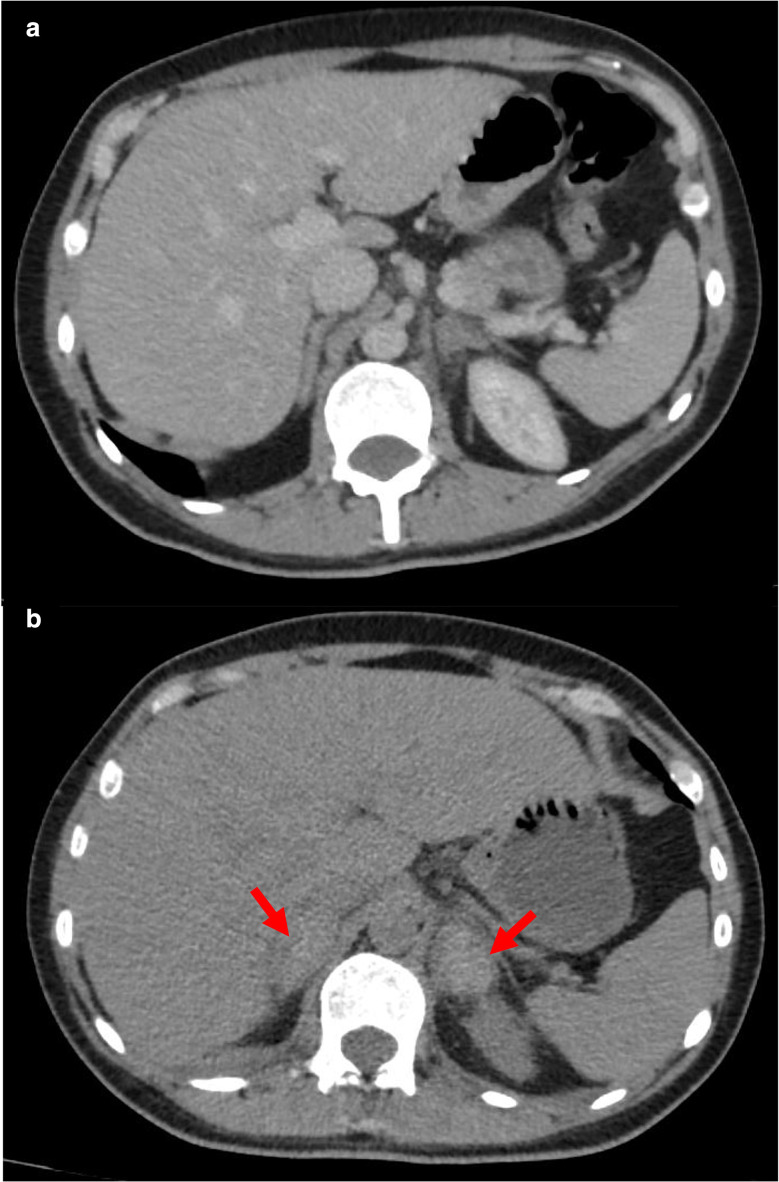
(A) Initial axial unenhanced CT scan of the abdomen on day of admission showing relatively unremarkable adrenal glands. (B) Axial unenhanced CT of the abdomen showing bilateral adrenal haematomas (arrows) Day 5 post-admission.
